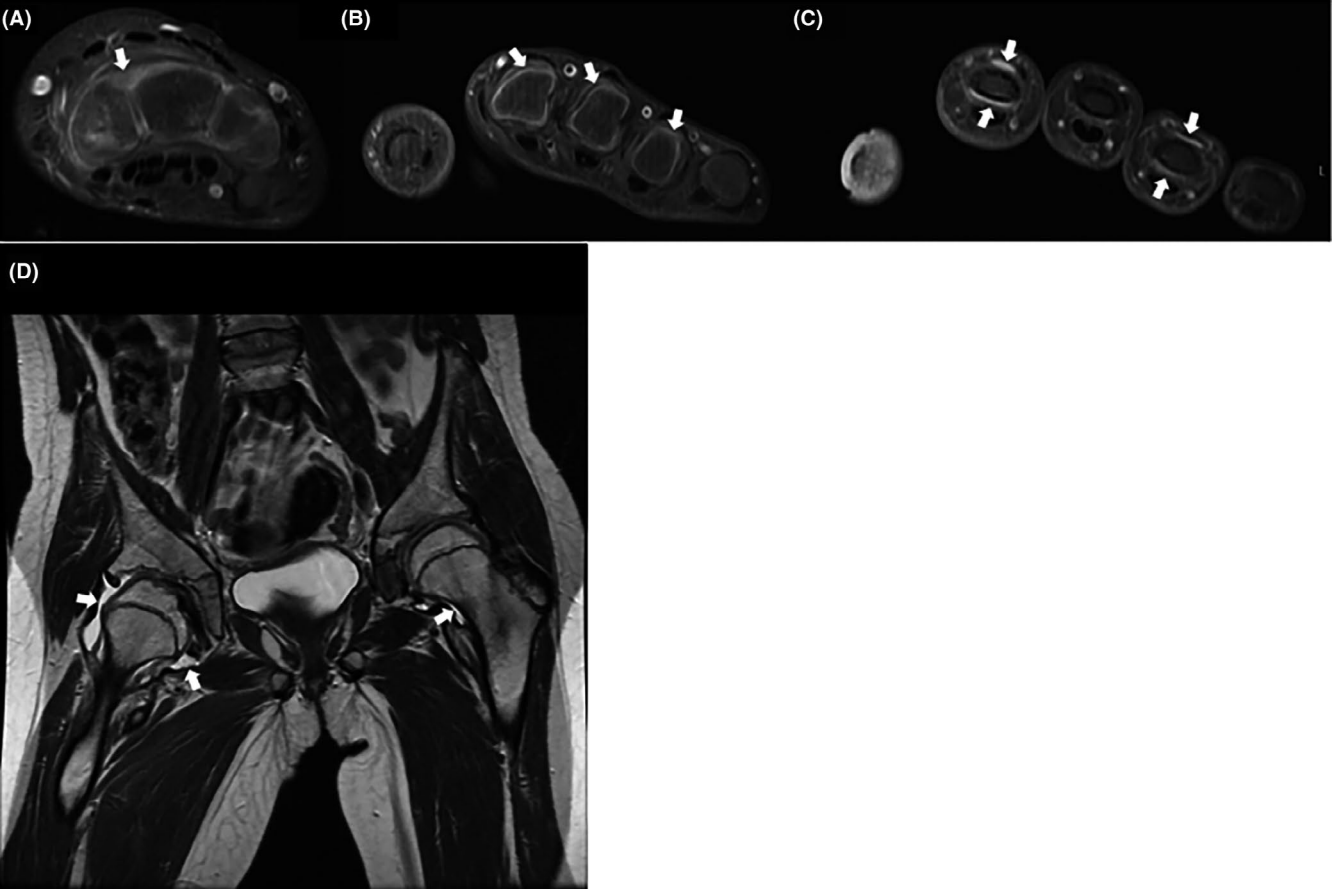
Axial contrast‐enhanced T1 fat‐saturated MR images show synovitis within the carpal joints (A), the MCP joints (B), and the PIP joints (C). (D) Coronal T2 MR image demonstrates large right hip and small left hip effusions, with irregularity of the right acetabulum and femoral head. MCP, metacarpophalangeal, PIP, proximal interphalangeal. Arrows indicate areas of synovitis
